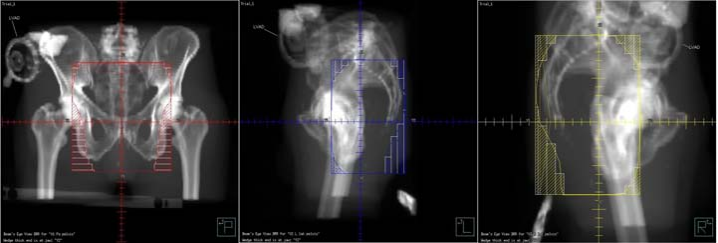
Digitally reconstructed radiographs (DRRs) of the patient's treatment fields with the LVAD pump identified. From left to right, the views are posterior to anterior, left lateral, and right lateral.
